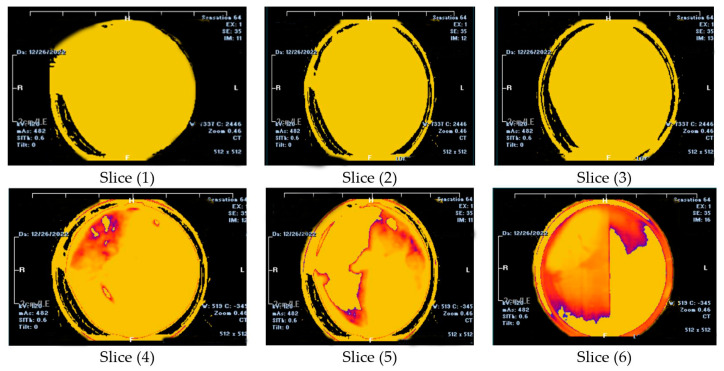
The CT Scan images for the mud cake samples containing 0.92 wt.% MNPs. The CT scan images were taken in the direction of the filtrate invasion. Slice 1 represents the top surface of the mud cake, whereas slice 6 represents the bottom surface.
